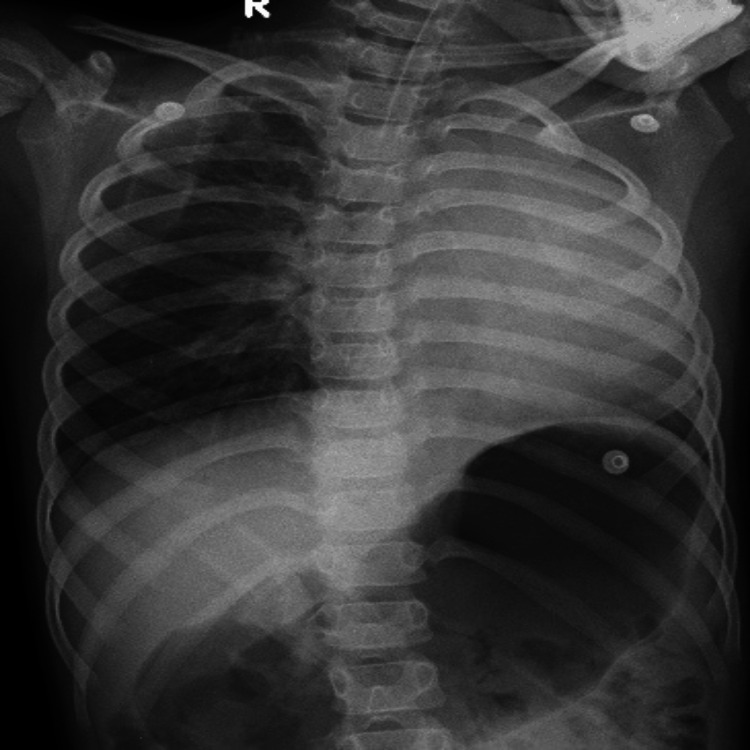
Chest Radiograph Demonstrating Left Lung Volume Loss Following Foreign Body Aspiration Chest radiograph obtained at presentation demonstrating left lung volume loss with compensatory hyperinflation of the contralateral lung following aspiration of a toy whistle component.
